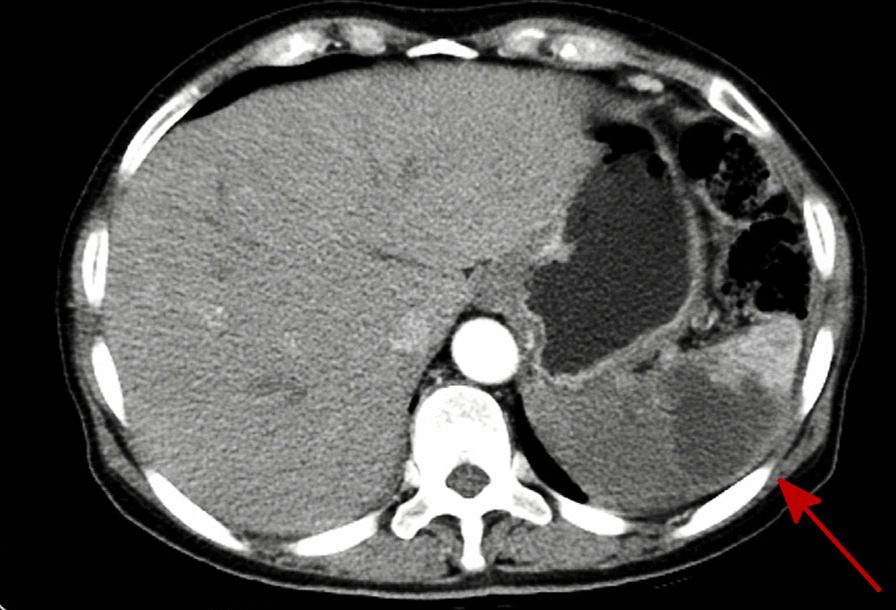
Abdominal computed tomography (CT) imaging revealed patchy hypodense shadow of the spleen with wedge-shaped changes
